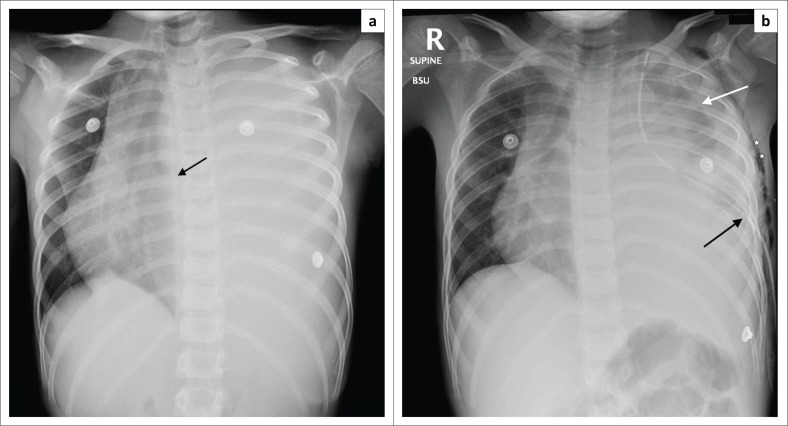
Frontal chest radiograph (CXR): (a) Initial CXR on presentation demonstrates complete opacification of the left hemithorax with resultant mediastinal shift to the right and cut-off of the left main bronchus (black arrow); (b) CXR post left chest wall/pleural biopsy. Left pneumothorax (white arrow) with the left intercostal drain in-situ (black arrow). Associated surgical emphysema of the left chest wall (white stars).
